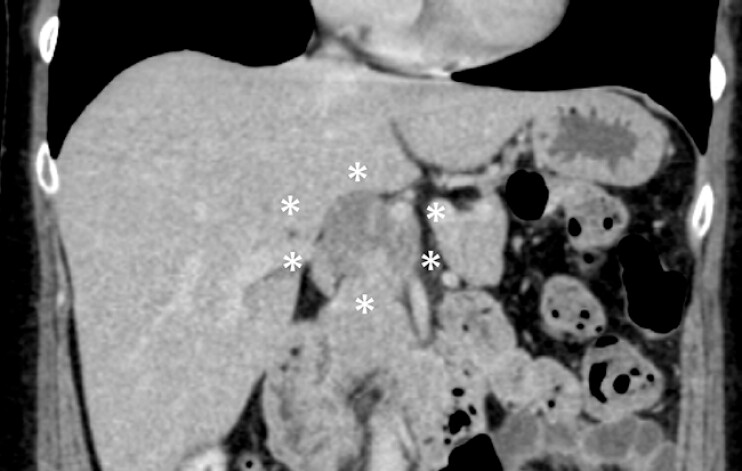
Follow-up contrast-enhanced CT at 3 months following initial presentation. Delayed-phase imaging showing a slight reduction in tumor size, with faint linear enhancement observed along the tumor margin and within the lesion (asterisk). The patient remained asymptomatic without any recurrent abdominal pain during follow-up. CT, computed tomography.
